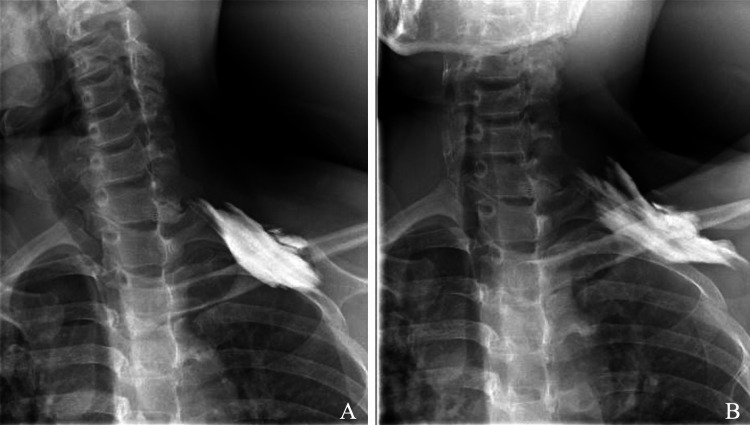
Radiographs after ultrasound-guided neurography of the brachial plexus. The contrast has moved caudally from the injection site, but the head side and head down positions show a blocked image (A). Upon left upper extremity elevation, which reduced symptoms, contrast migration to the head side is observed (B).
